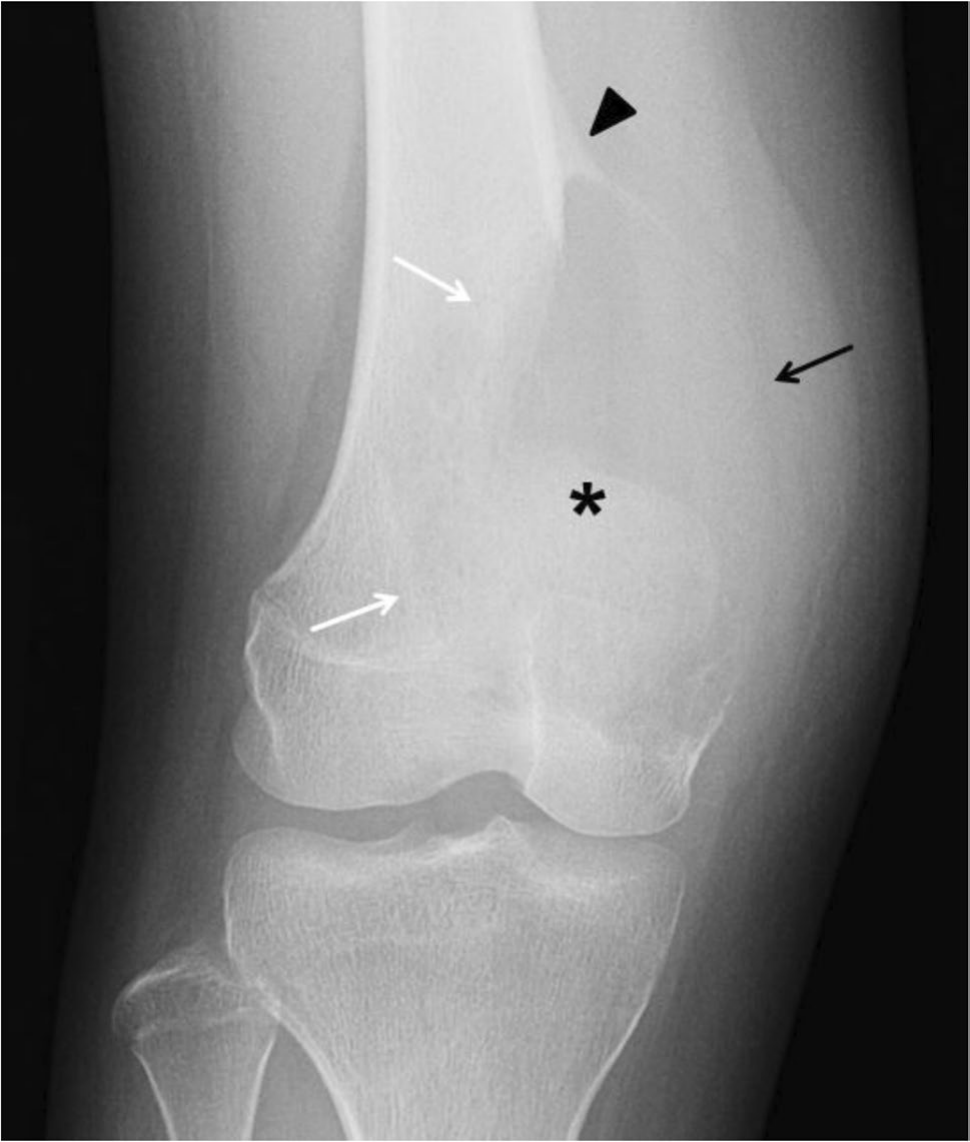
Radiographically aggressive, biopsy proven aneurysmal bone cyst (ABC) of the distal femur in a 17-year-old boy (compare the striking similarity with patient from Fig. 10). An anteroposterior radiograph of the distal left femur shows a lytic distal metadiaphyseal lesion (*asterisk*) of the left femur. The lesion extends into the epiphysis and adjacent soft tissues with no calcified peripheral shell (*black arrow*). The ABC has a wide zone of transition (*white arrows*) and aggressive periosteal reaction proximally (*arrowhead*). The lesion was biopsied twice and ABC was confirmed twice
